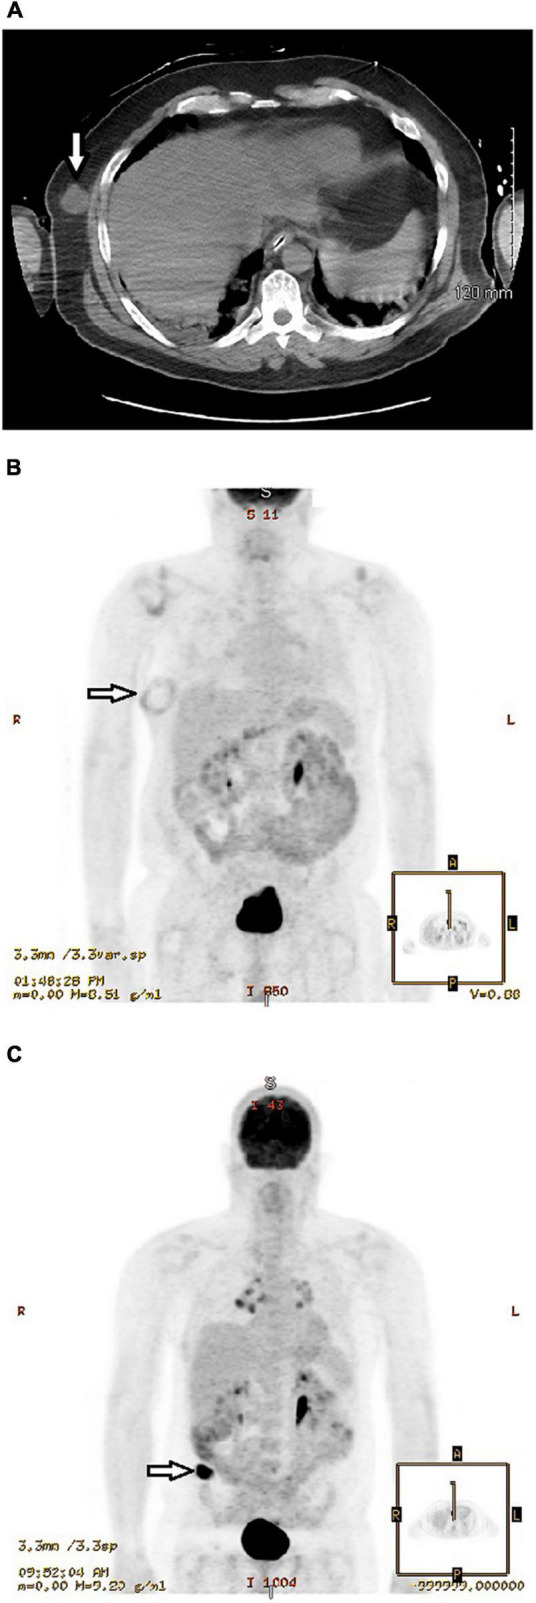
(A) Initial CT scan of chest without contrast performed 27 July 2020 showed extensive patchy ground-glass infiltrates throughout both lungs and an incidental 2.4 cm diameter nodular mass (arrow) in the right lateral lower chest wall of indeterminate significance and etiology. He underwent excision of the nodule on 23 October 2020. (B) Positron emission tomography (PET) scan performed 30 October 2020, 1 week after excision of the soft tissue nodule, showed a rounded mass that measured up to 6.7 cm with a rim of abnormal fluorodeoxyglucose (FDG) activity surrounding the mass (arrow). The enlargement was attributed in part to a hematoma that developed after the initial excision. However, a wide re-excision on 23 November 2020 showed that the melanoma had spread to the inked resection margins with positive lymph nodes containing metastatic melanoma in the subcutaneous adipose tissue and tumor nodules in the deeper subcutaneous tissue. (C) Positron emission tomography (PET) scan performed 27 August 2021 showed a hypermetabolic mass in the subcutaneous fat of the right buttocks region (arrow) that measured 4.7 × 2.2 cm. In comparison, PET scan on 21 May 2021, approximately 3 months earlier, showed no hypermetabolic masses or other areas of abnormal increased glucose metabolism. Biopsy of the mass confirmed malignant melanoma.
